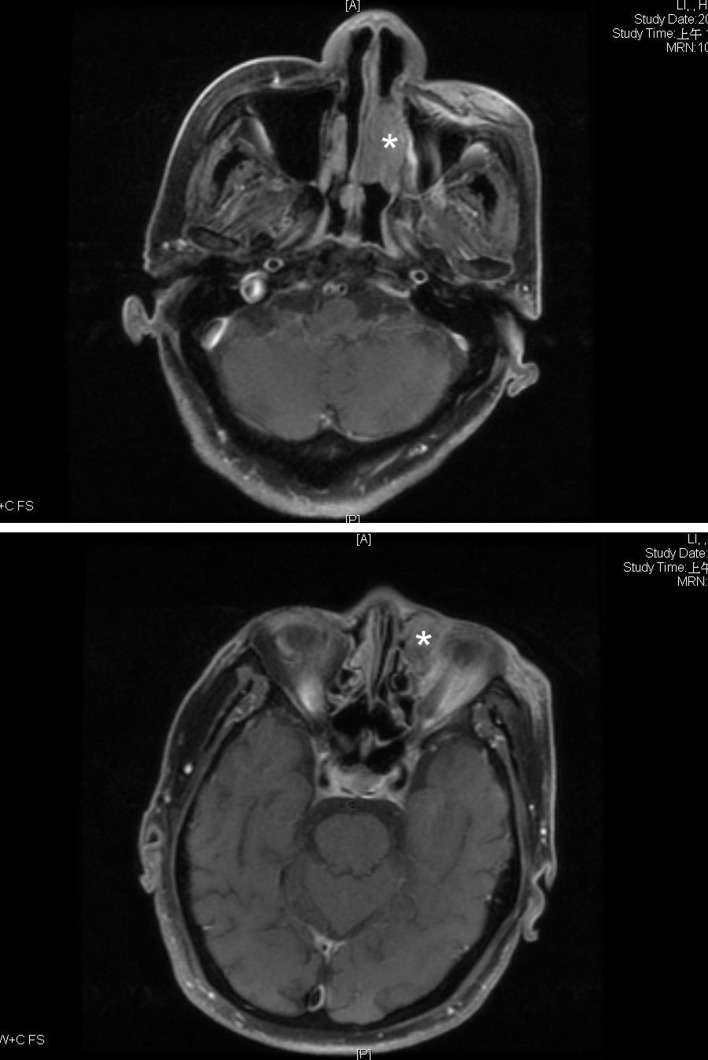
Contrast enhanced T1-weighted MRI showed (A) an enlarged hyperdense lesion in left nasal cavity (asterisk), (B) A hyperdense lesion in left medial aspect of orbit with involvement of preseptal space and retrobulbar fat were found on (asterisk).
